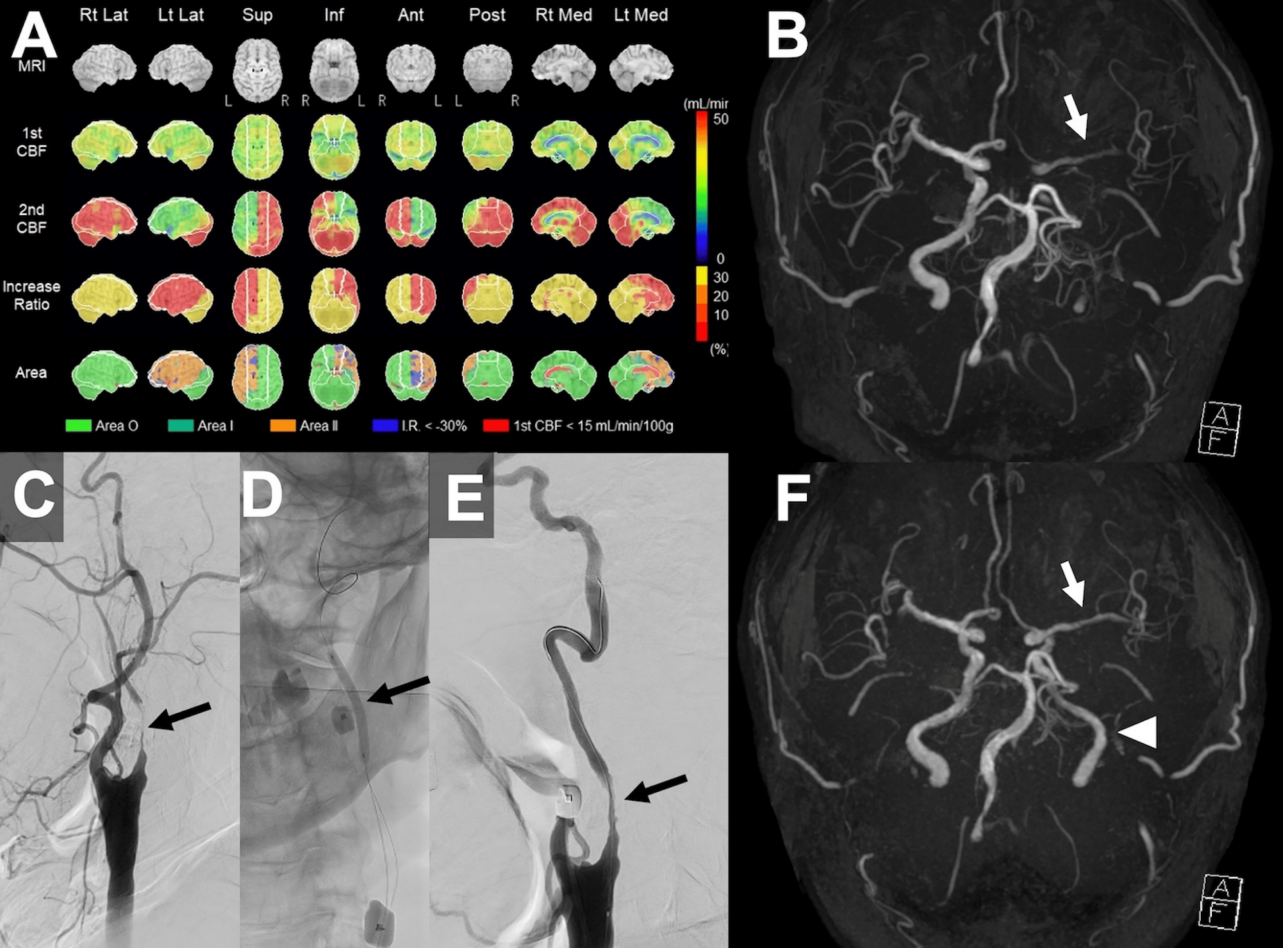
Preoperative perfusion imaging, initial angioplasty, and sequential magnetic resonance angiography. (A) Iodine-123 N-isopropyl-p-iodoamphetamine single-photon emission computed tomography (IMP-SPECT) demonstrating decreased cerebral perfusion and impaired vascular reserve in the left hemisphere. (B) Time-of-flight magnetic resonance angiography (TOF-MRA) showing attenuated signal intensity in the left middle cerebral artery compared to the contralateral side. (C) Digital subtraction angiography of the left carotid artery revealing high-grade stenosis of the internal carotid artery. (D) Endovascular intervention with percutaneous transluminal angioplasty (PTA) performed under proximal protection. (E) Post-interventional angiogram showing modest luminal gain at the site of stenosis. (F) Post-interventional TOF-MRA depicting improved flow signal in the left internal carotid artery (arrowhead) and middle cerebral artery (arrow).
